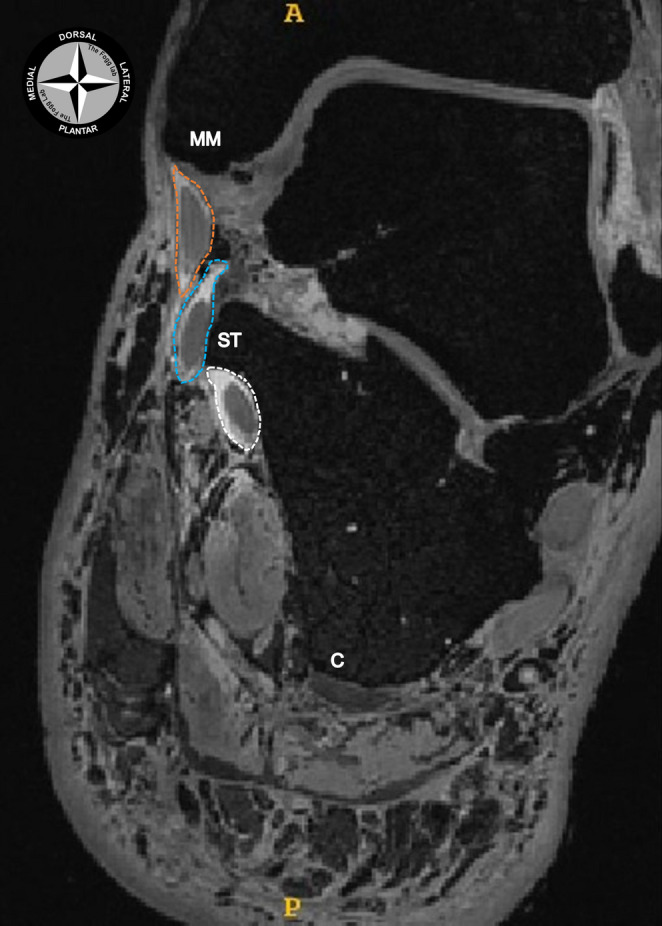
A coronal section of an MR image at the level of the tarsal tunnel. The tendinous sheaths of the tibialis posterior (orange dotted line), flexor digitorum longus (blue dotted circle) and flexor hallucis longus (white dotted line) tendons were injected with Magnevist. This enhanced the visualisation of the separate tendinous sheaths at the level of the sustentaculum tali (ST) within the tarsal tunnel. MM: medial malleolus; C: calcaneus
